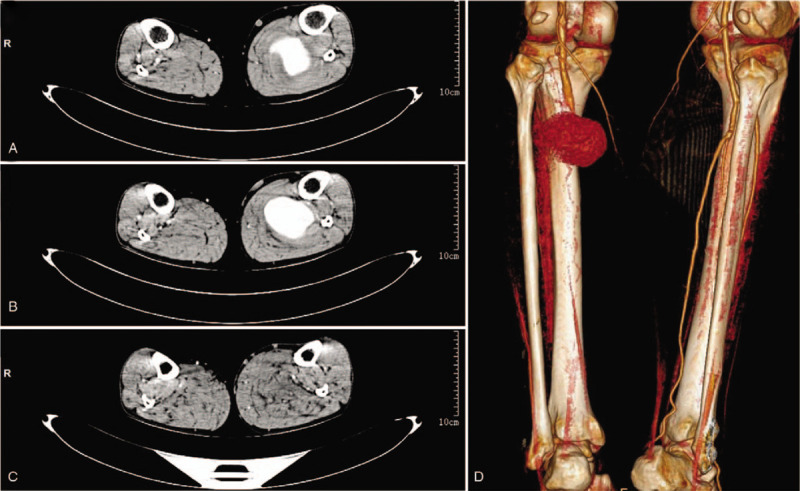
(A) and (B) Axial CTA scans are revealing cross-section of the PSA and (C) the swollen left shank. (D) Three-dimensional reconstruction image demonstrates the volume of PSA. CTA = computed tomographic angiography, PSA = pseudoaneurysm.
